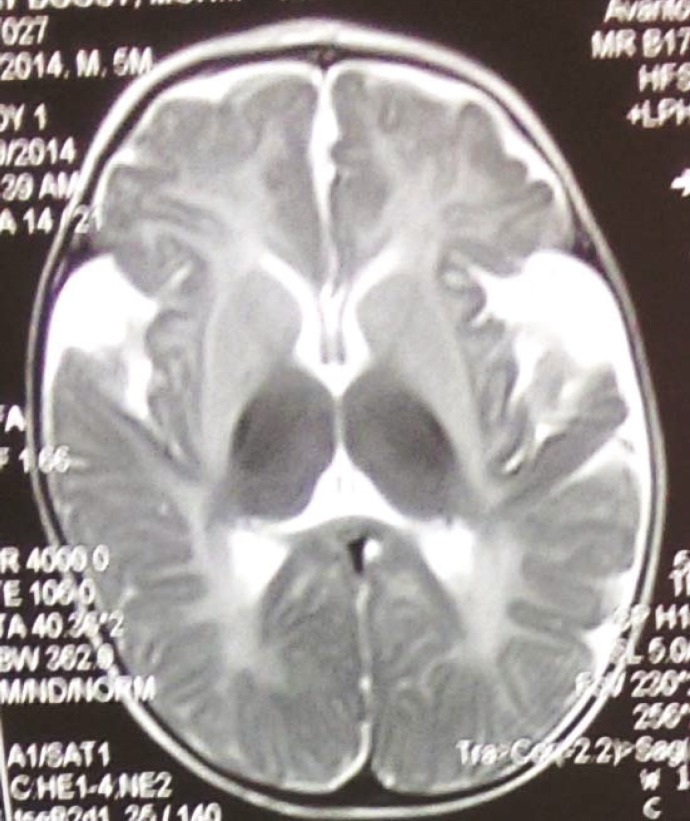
A 6-month-old boy-with Glutaric aciduria type1 with sylvian fissure widening in axial brain MRI
